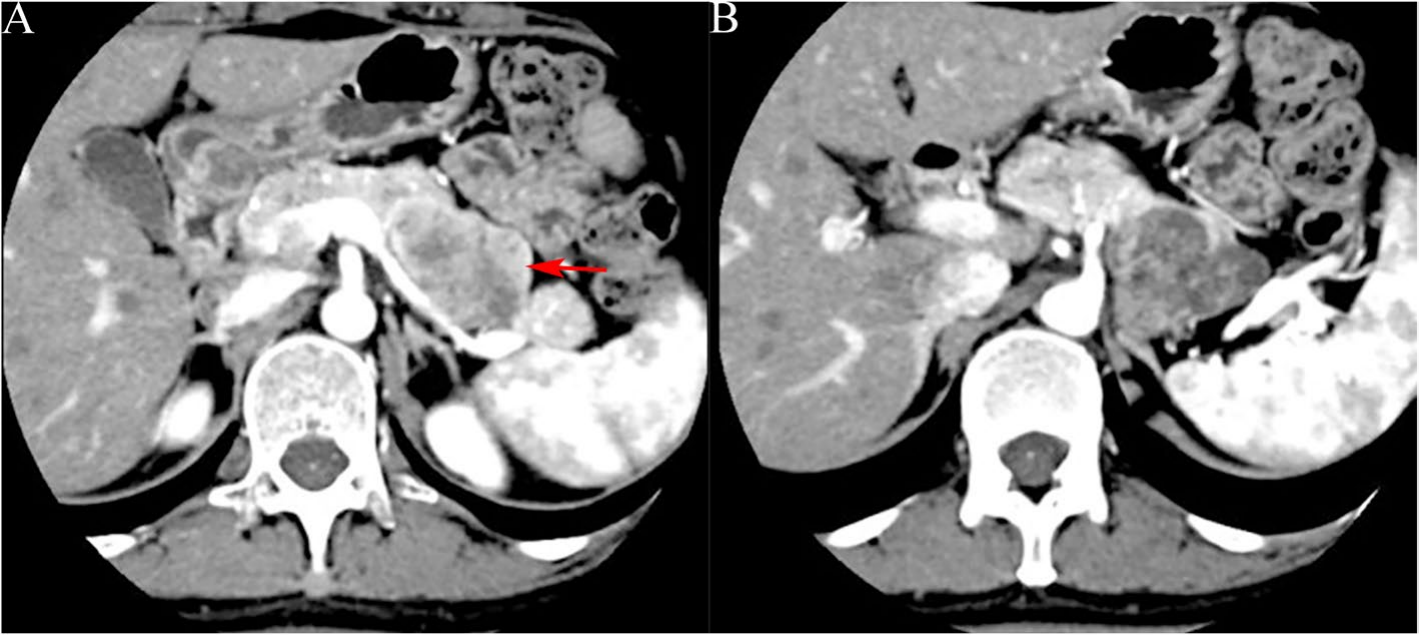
A 37-year-old male with solid pseudopapillary tumor. CT target scanning shows that (a) the tumor is located on the pancreatic body and tail and is triangle-like, the tumor capsule is complete and linearly enhanced obviously (long arrow) during the pancreatic parenchymal phase; (b) the lower margin of the tumor is lobulated and grows out of the pancreas during the pancreatic parenchymal phase
